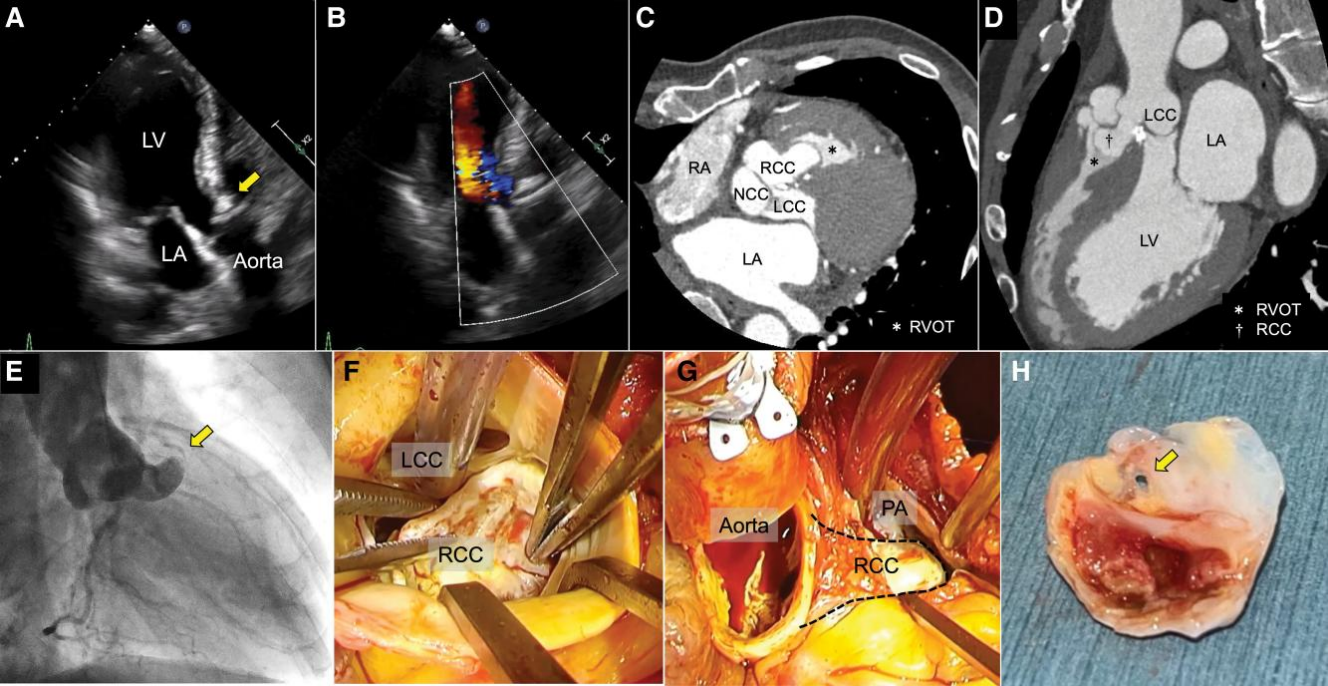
(*A* and *B*) Transthoracic echocardiography demonstrated the severe calcified right coronary cusp (arrowhead) and moderate aortic regurgitation. The diameter at the level of the sinus of Valsalva was 36 mm. Contrast-enhanced computed tomography axial view (*C*) and left anterior oblique view (*D*) showing the deformed right coronary cusp reached the right ventricular outflow tract. (*E*) Aortography demonstrated the deformed right coronary cusp with a shunt flow to the right ventricular outflow tract (arrowhead). (*F*) The aortic valve was a tricuspid, and the right coronary cusp was enlarged and calcified and had limited mobility. (*G*) The right coronary cusp was deformed, lodged into the ventricular septum, and reached the right ventricular outflow tract. (*H*) The resected right coronary cusp shows a pin-hole (arrowhead). RCC, right coronary cusp; LCC, left coronary cusp; NCC, non-coronary cusp; RA, right atrium; LA, left atrium; LV, left ventricle; RVOT, right ventricular outflow tract. ＊Right ventricular outflow tract; †right coronary cusp.
